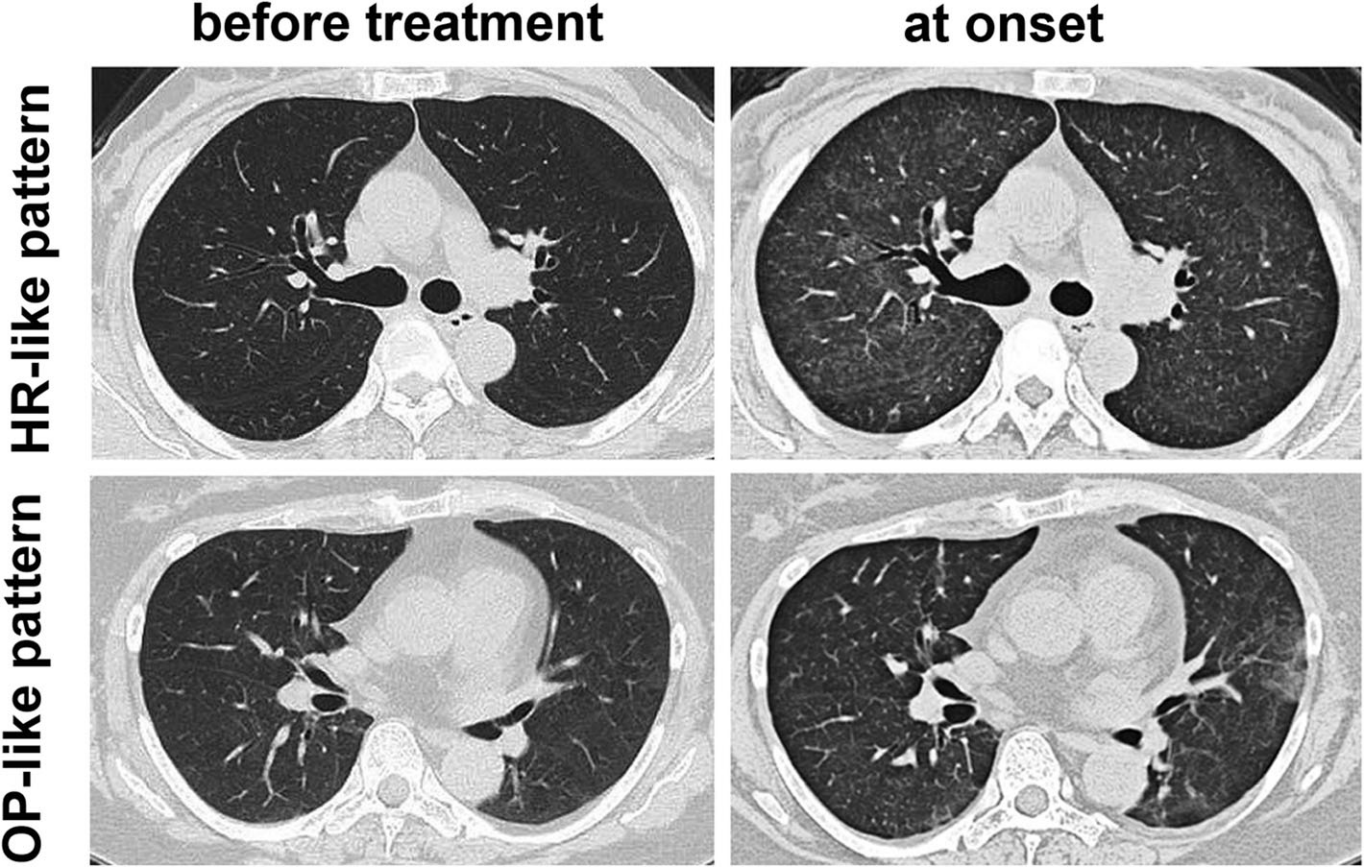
Computed tomography of the chest before neoadjuvant chemotherapy and at the onset of interstitial lung disease in a 63-year-old female (with a hypersensitivity reaction-like pattern similar to that seen in case number 3) and in a 57-year-old female (with an organizing pneumonia-like pattern similar to that seen in case number 4)
